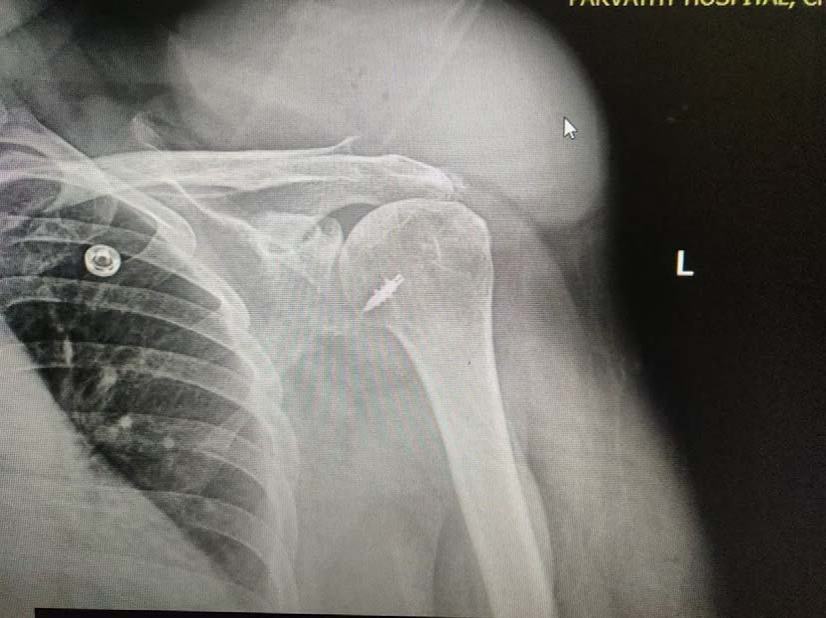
X-ray of the affected shoulder showing destruction of AC joint and metal anchor in-situ.
